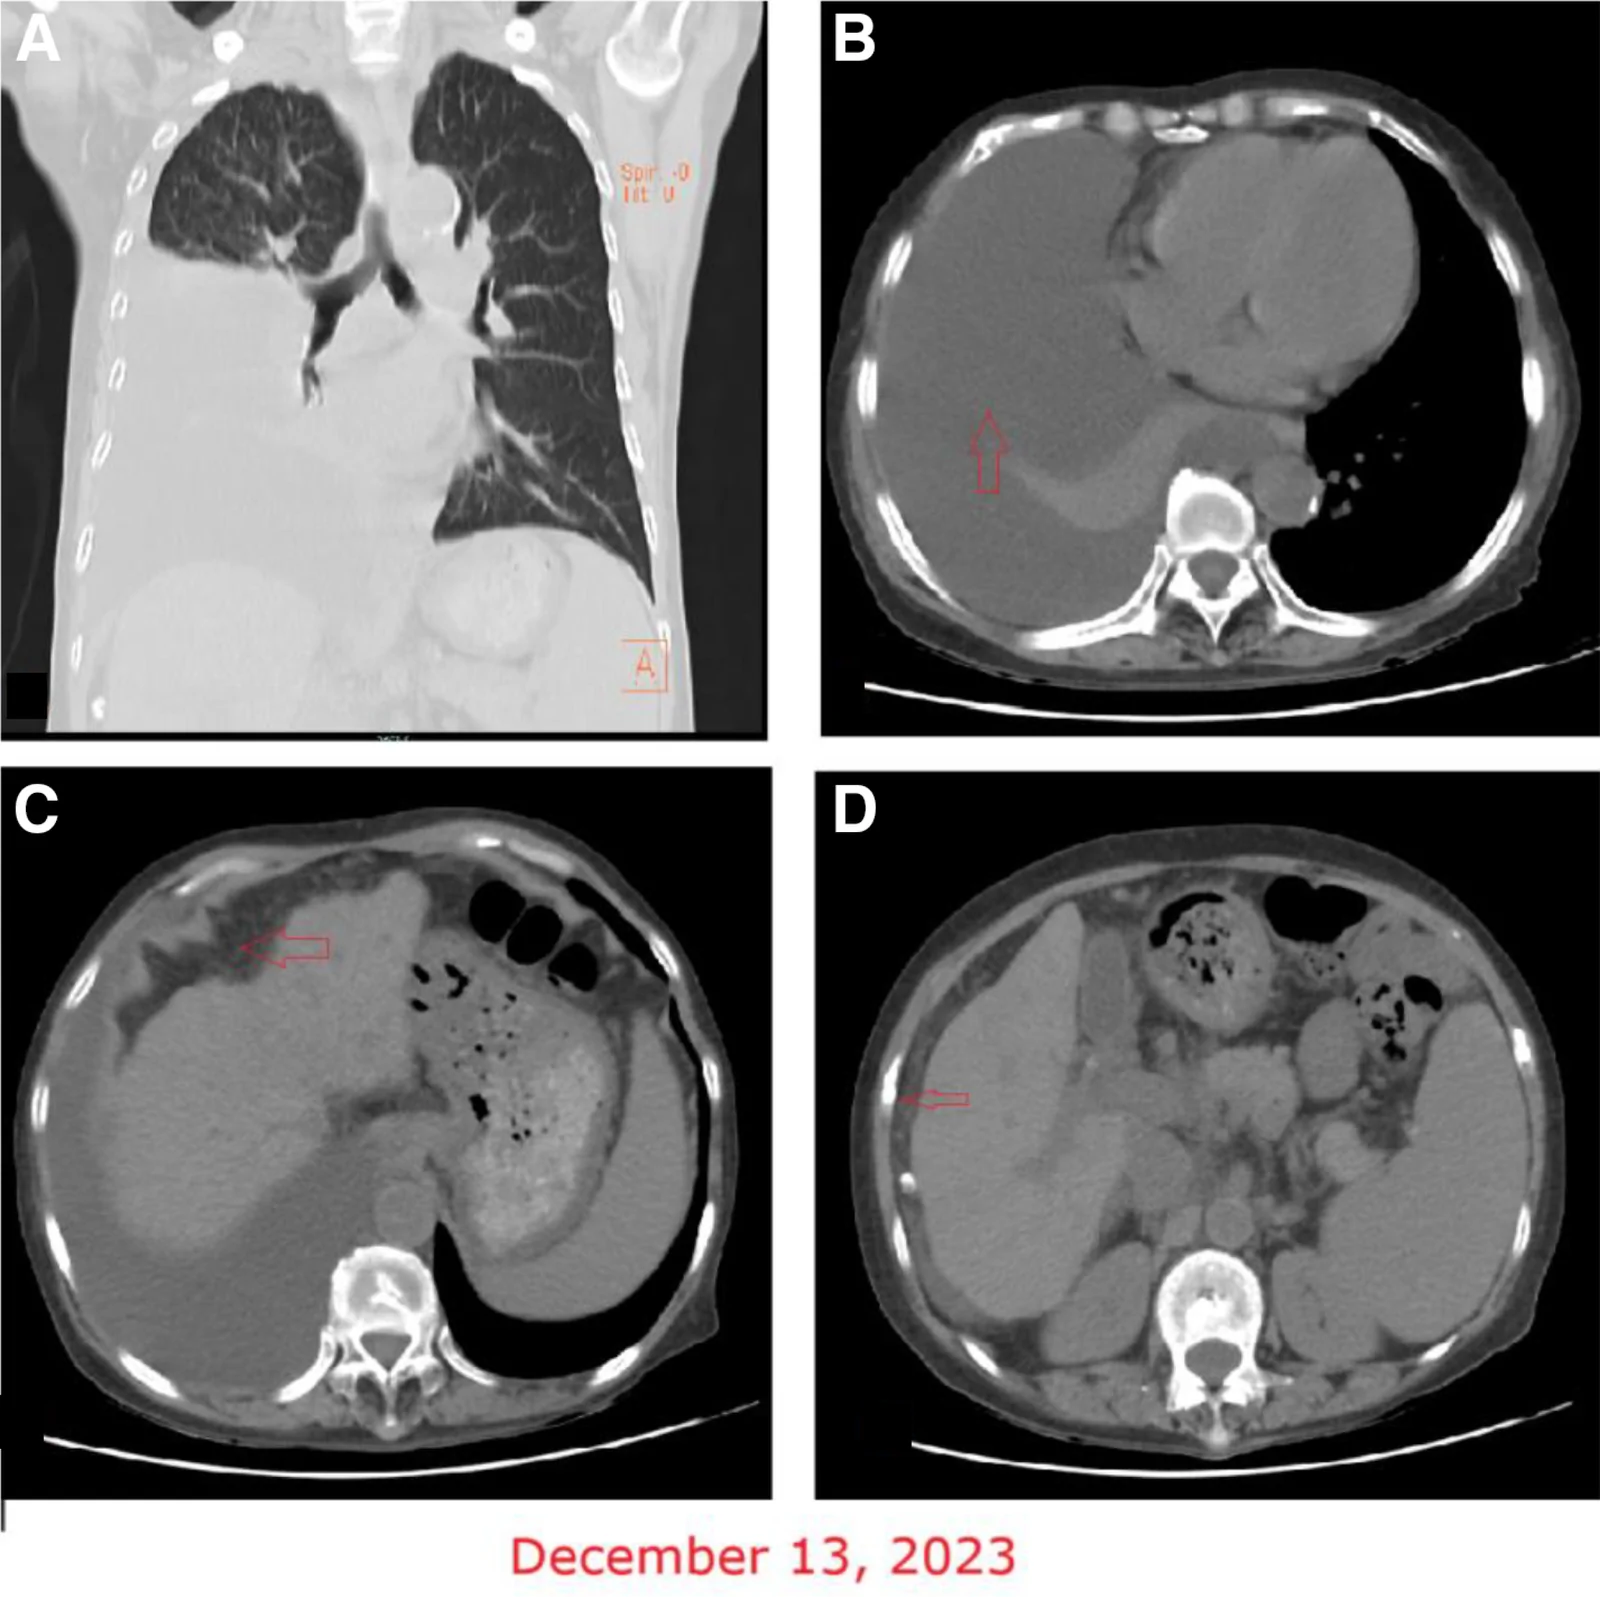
Pulmonary CT imaging findings of hepatic pleural effusion. (A): In the right thoracic cavity, there was large right pleural effusion with passive atelectasis, accompanied by inferior displacement of the right diaphragm and superior displacement of the left diaphragm.(B): The right lower lobe was fully compressed, and there was no ventilation within the lung.(C): The right diaphragmatic folds exhibited variable thicknesses, and some formed a 1-way valve-like structure connecting the abdominal cavity to the thoracic cavity. The liver surface was irregular, with poor adhesion to the diaphragm. (D): There was no notable ascites in the abdominal cavity, and only minimal perihepatic ascites was detected. CT = computed tomography.
